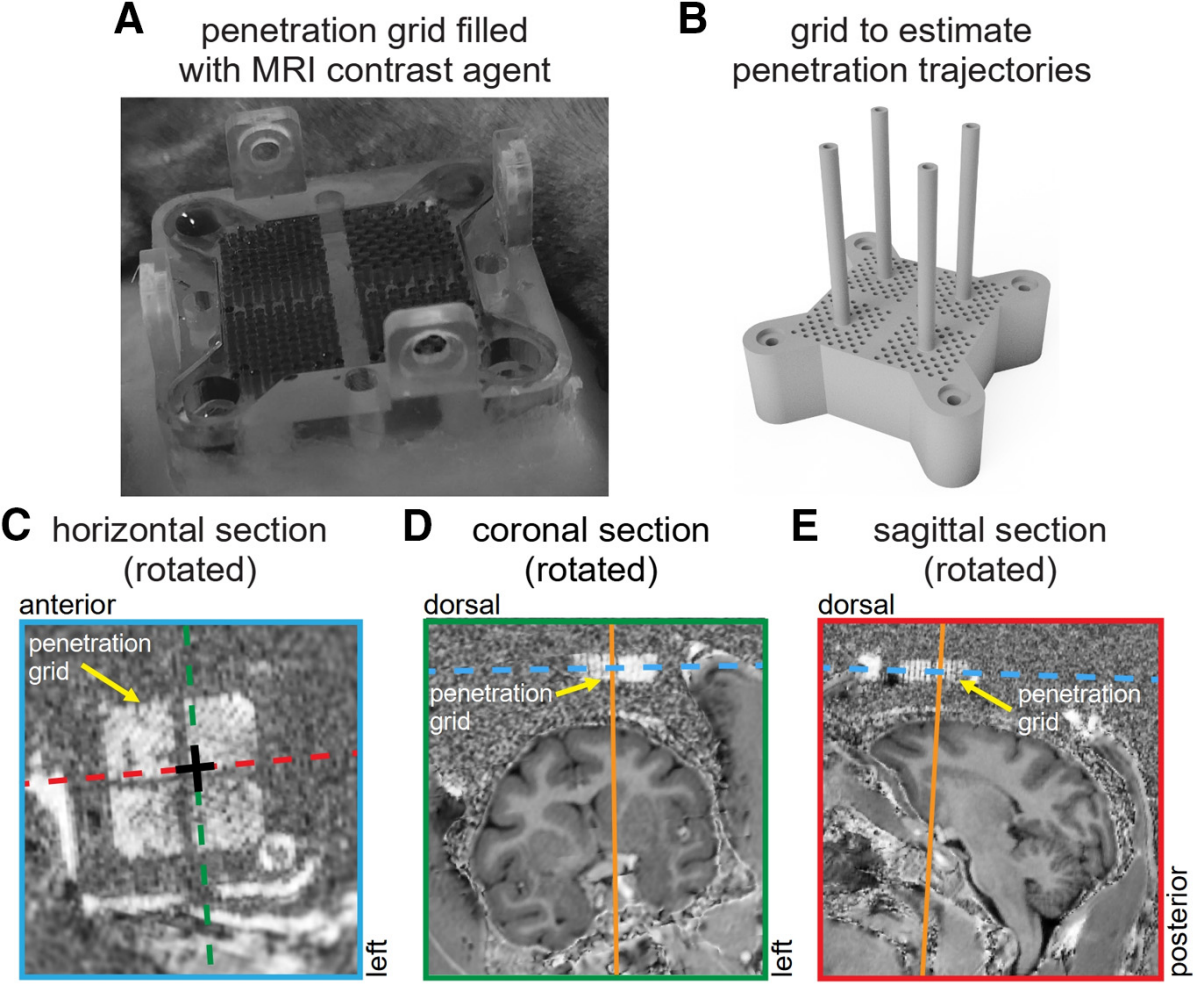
Estimating trajectories using the penetration grid. *A*, Picture of the penetration grid secured to the implant and filled with an MRI contrast agent (povidone-iodine ointment). *B*, 3D rendering of a specialized grid for making precise estimates of penetration trajectories. This grid has the same pattern of penetration holes as the standard penetration grid (*A*), but is thicker (10 mm, compared to 5 mm) and has four hollow pillars which are perpendicular to the grid surface for filling with an MRI contrast agent. These features can aid in the estimation of penetration trajectories. *C–E*, Rotated MRI sections aligned relative to the horizontal plane of the penetration grid shown in *A*. *C*, Rotated horizontal MRI section providing a top-down view of the penetration grid. The four quadrants of the grid are visible and the penetration hole used in testing the microdrive is marked (black crosshair) along with the rotated coronal (green dashed line) and rotated sagittal (red dashed line) MRI sections. *D*, Rotated coronal MRI section aligned relative to the horizontal plane defined by the penetration grid (blue dashed line), showing the estimated penetration trajectory (orange line). Note that the penetration trajectory is perpendicular to the horizontal plane of the penetration grid. *E*, Same as *D* but for the rotated sagittal MRI section.
